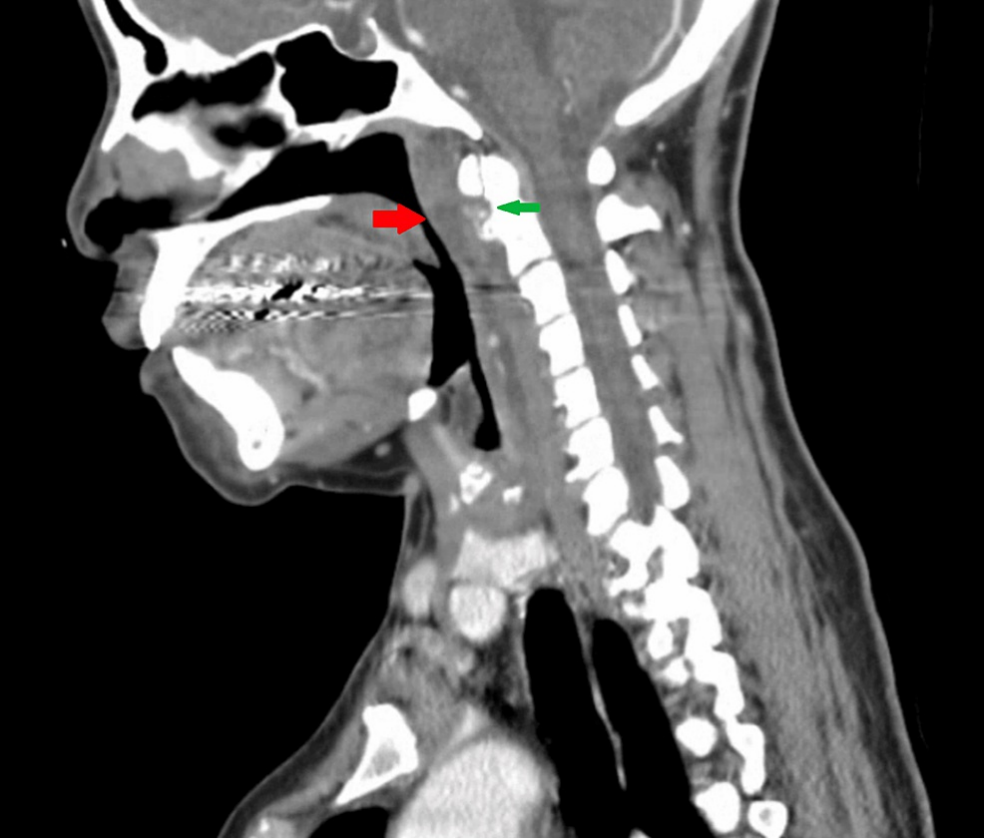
Contrast-enhanced computed tomography (CT) of the neck (sagittal view) Retropharyngeal effusion extending from C1 to C6 (red arrow); Amorphous calcification of the superior fibers of the longus colli tendons (green arrow)
